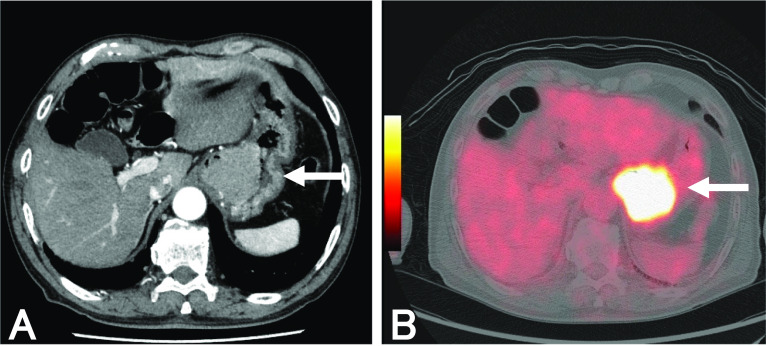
Image findings. A Computed tomography. Computed tomography reveals a mass, 50 mm in diameter, in the EGJ (arrow). B
